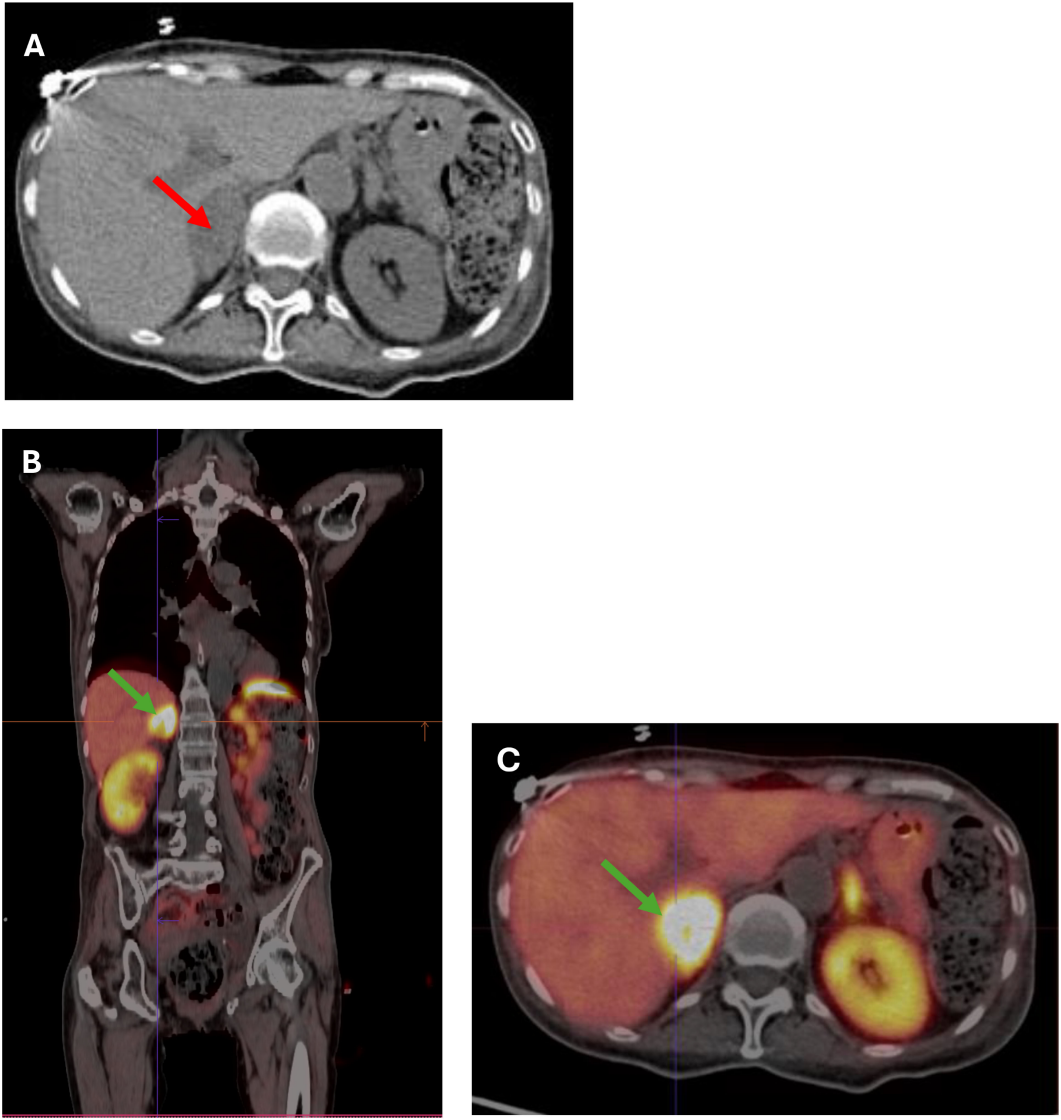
(A) CT scan showing a 32x35 mm heterogeneous, well-demarcated litho-cystic lesion (red arrow) in the right adrenal gland. (B, C) (B) Whole-body [68Ga] DOTATATE PET-CT image showing a hypodense right adrenal nodule measuring 36x22 mm (SUV max 35.4) (green arrow). (C) Cross-section [68Ga]Ga-DOTA-TATE PET/CT image showing a hypodense right adrenal nodule 36x22 mm (SUV max 35.4) (green arrow).
